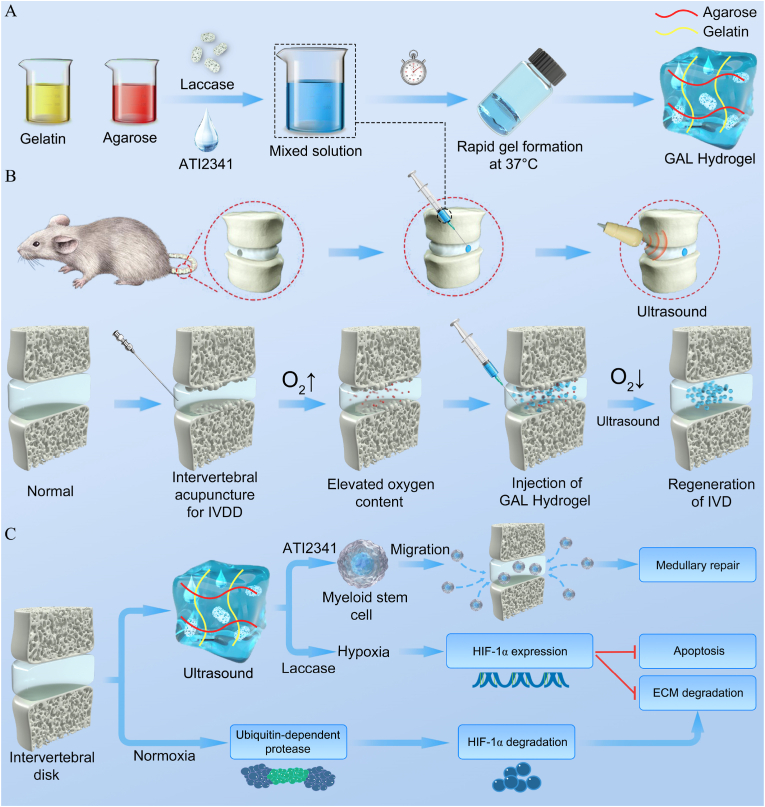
Preparation scheme and therapeutic mechanism of Gelatin-Agarose-Laccase (GAL)-ultrasound responsive hydrogel. (A) Construction of GAL hydrogels. (B) GAL hydrogel was injected into the intervertebral disc to induce a hypoxic microenvironment and synergized with ultrasound for intervertebral disc degeneration (IVDD) treatment. (C) Ultrasound-mediated improvement of IVDD by GAL hydrogel and the underlying mechanism.
